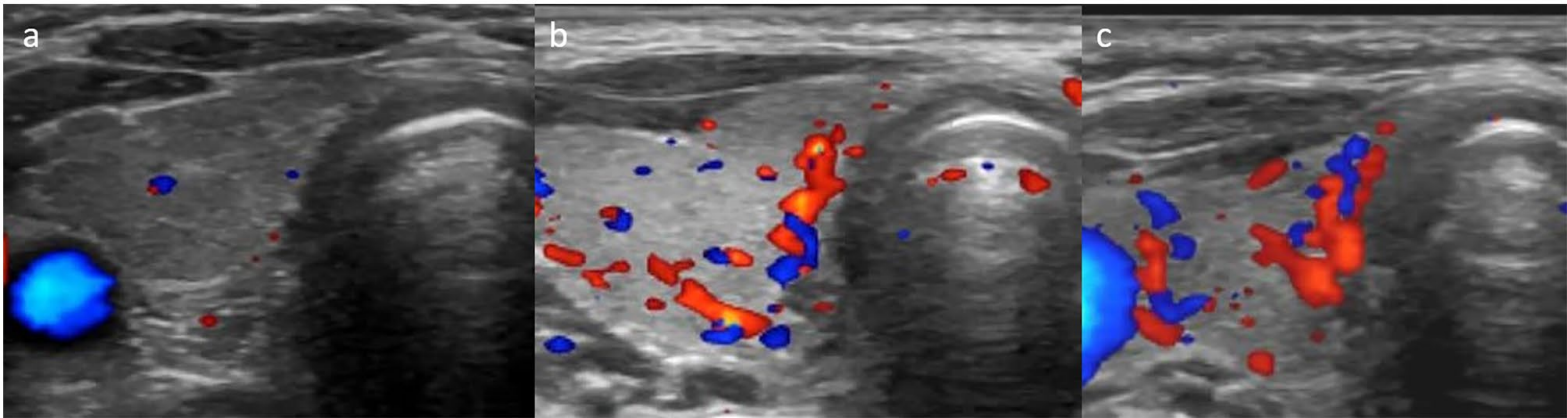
Examples of color Doppler ultrasonography patterns of patients; (a) patient number 10-pattern 0; (b) patient number 7-pattern 1; patient number 11-pattern 2
